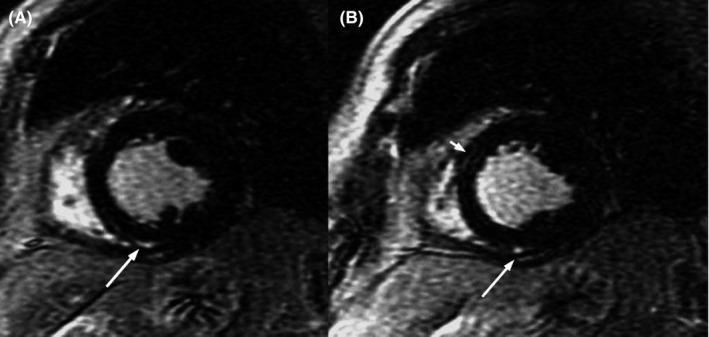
Short‐axis late gadolinium enhancement cardiac MR image showing intramyocardial late enhancement areas at the insertion of the posterior wall of the right ventricle on the interventricular septum (long arrow, panel A and B) and midmyocardial late enhancement area in the anterior interventricular septum at the base and middle level with non‐ischemic pattern indicating fibrosis (short arrow, panel B)
